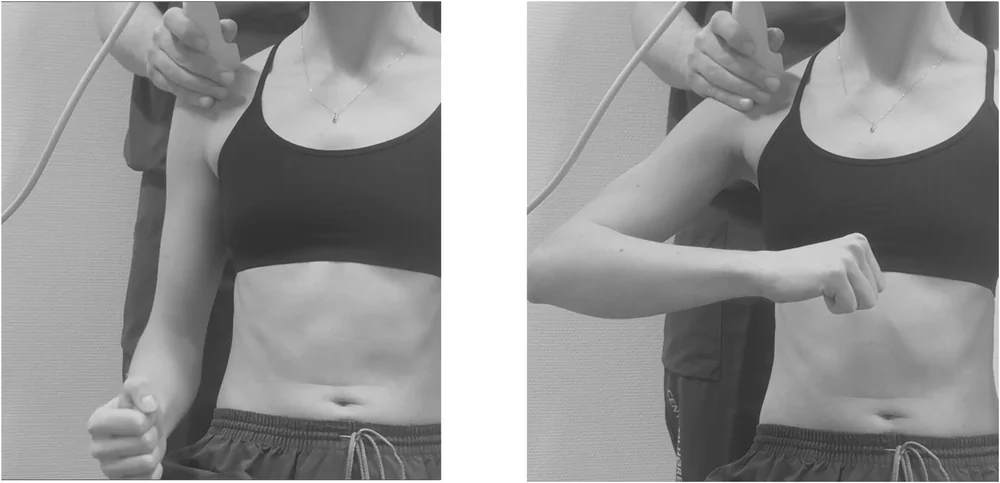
Evaluation of ultrasonographic impingement. Start position (left) and end position (right).
